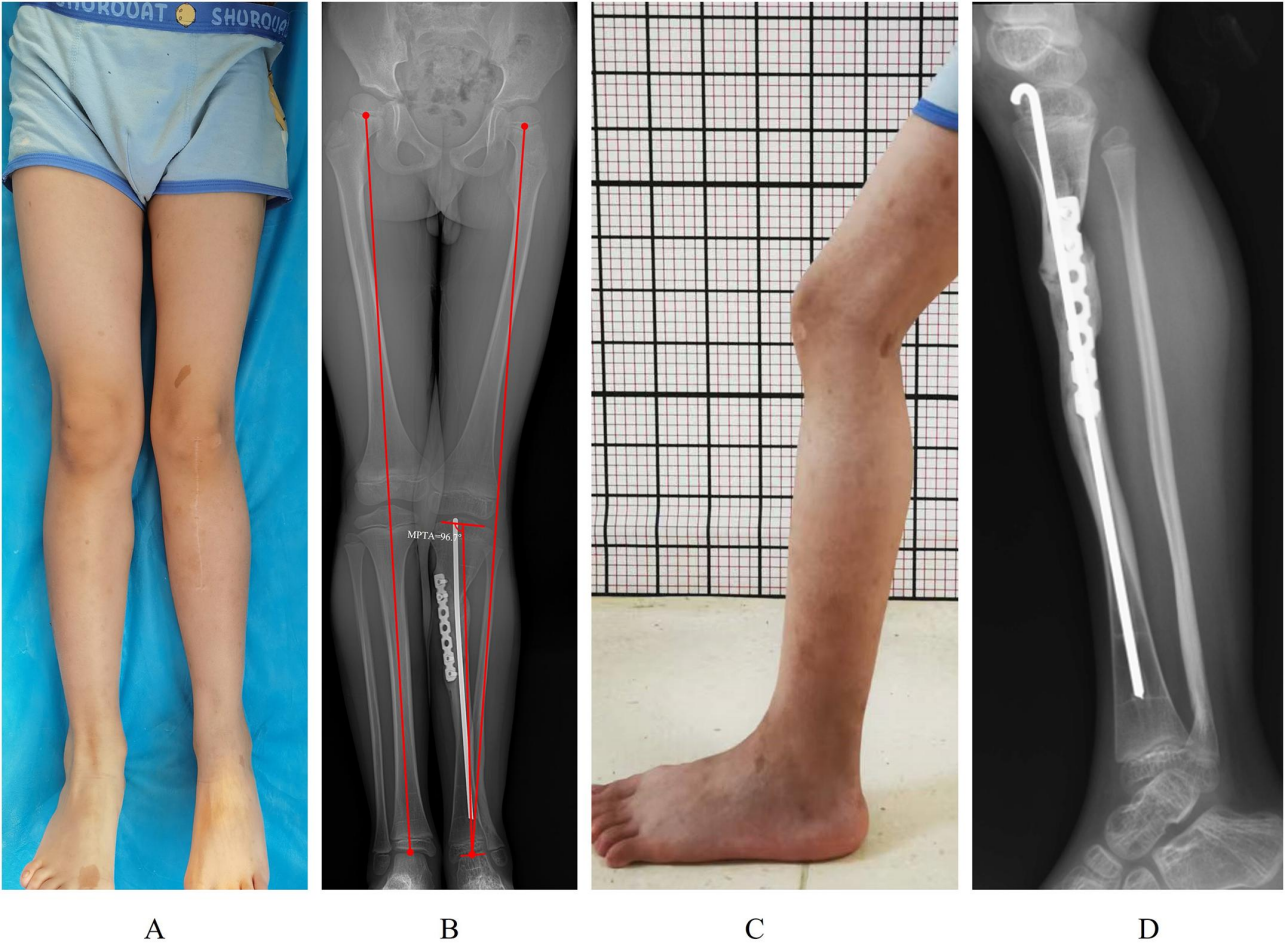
Lower limb appearance and radiographs of the child at final follow-up. (A) Anterior clinical photograph showing equal limb lengths and mild left genu valgum. (B) Full-length anteroposterior radiograph of both lower limbs showing left genu valgum and 0.6 cm of left-sided shortening. (C) Lateral clinical photograph demonstrating normal morphology of the left leg.
